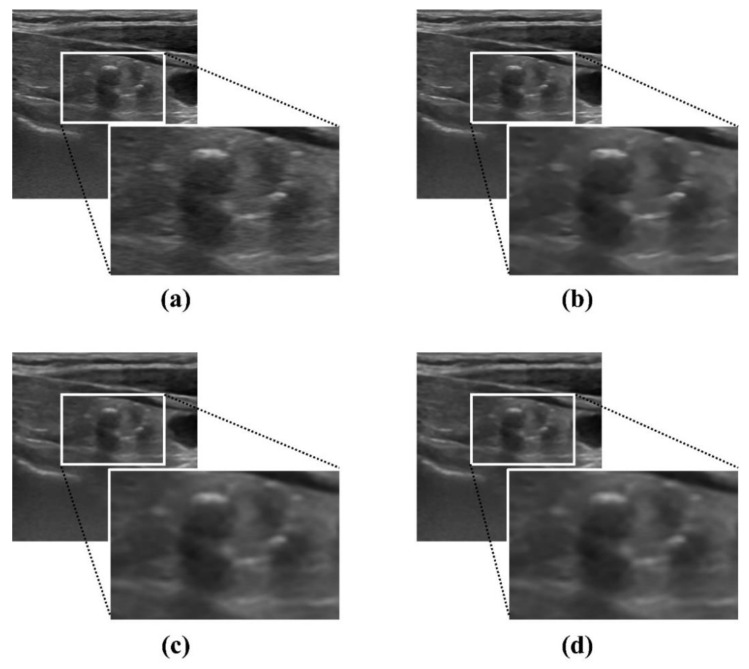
Ultrasound images of patients with thyroid nodule: (a) noisy image and NLM denoising with (b) low smoothing, (c) middle smoothing, and (d) high smoothing.
